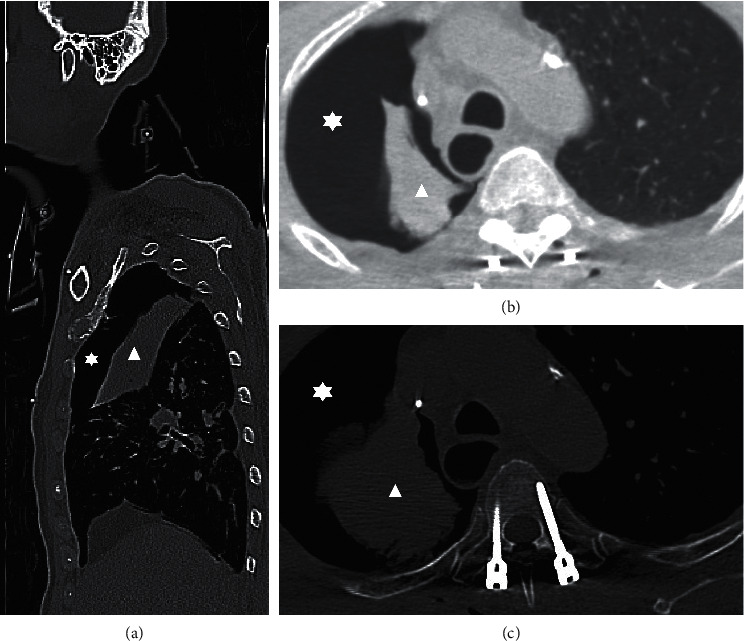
Postop immediate CT showed right apical lung pneumothorax (star) and consolidation at the right lung, leading to the radiologist's suspicion of tumor or collapsed lung (triangle): (a) sagittal view; (b) lung window.
